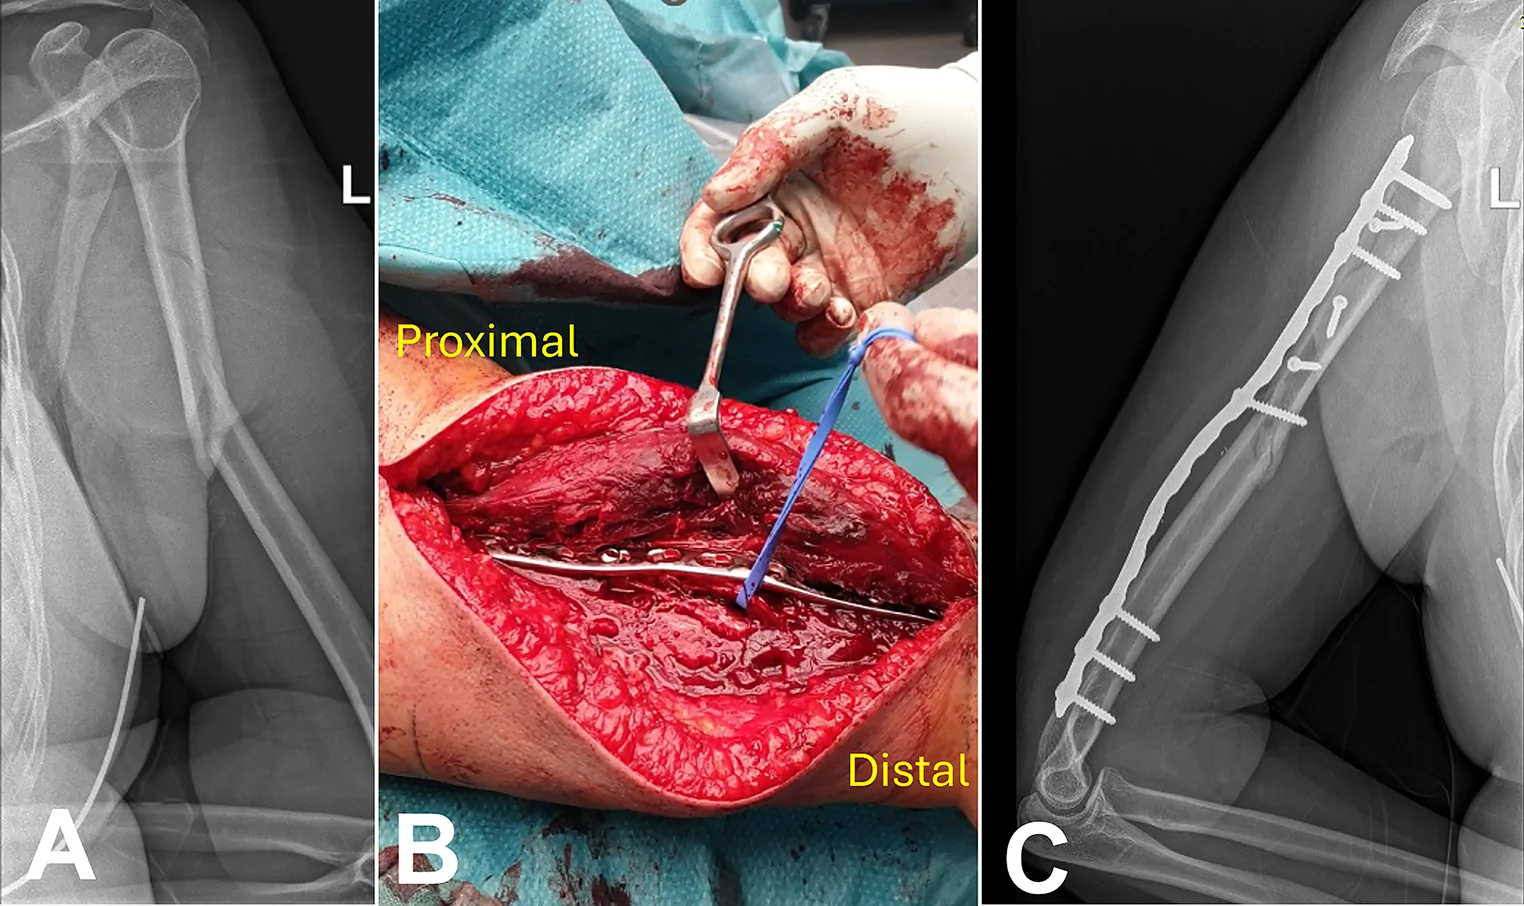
A: Preoperative x-ray of a spiral midshaft humerus fracture in a 50-year-old female. B: Intraoperative view of a posterior RNBP with the looped radial nerve underneath the plate at the wave segment. C: One month postoperative lateral radiograph view
